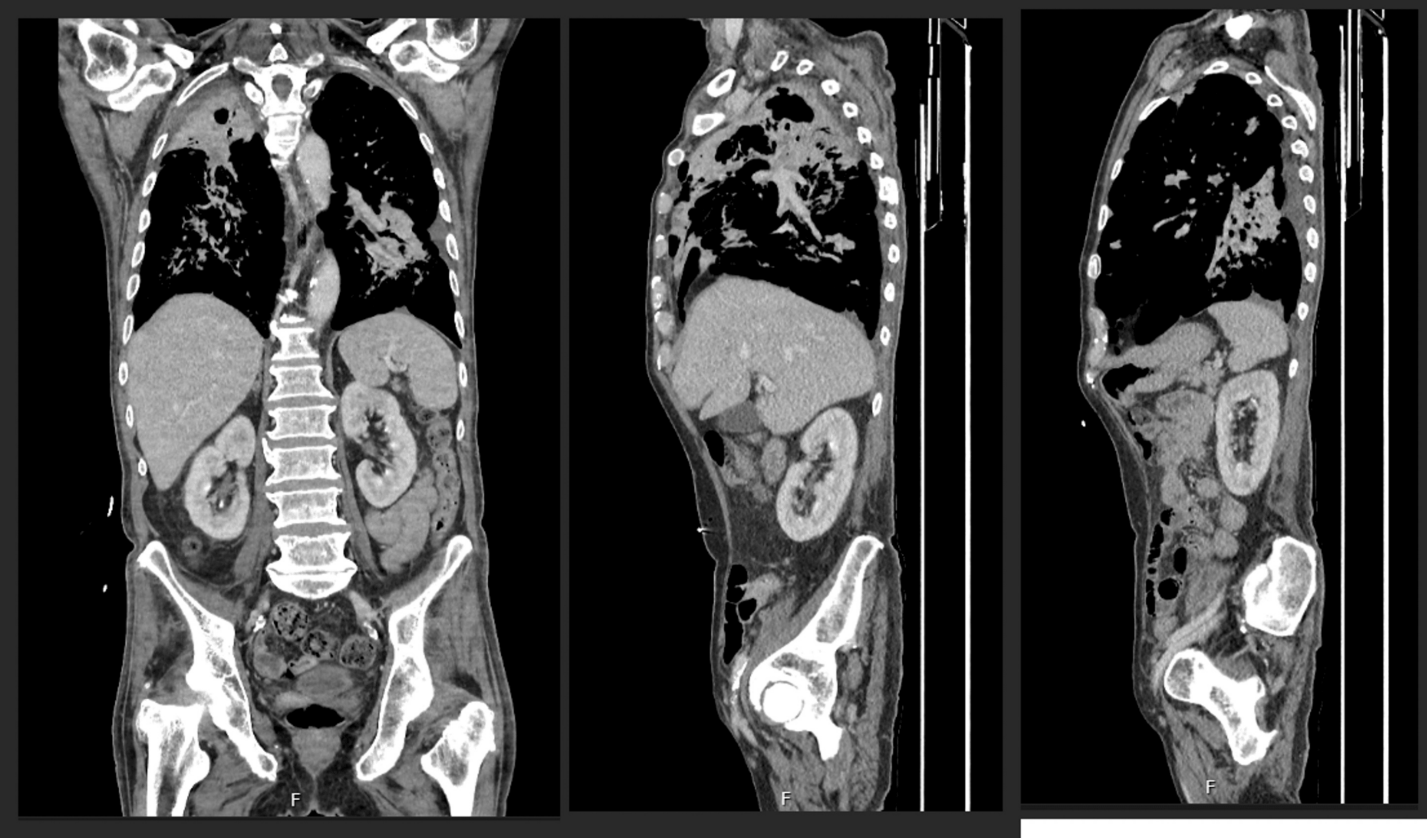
CT chest, abdomen, and pelvis showing stigmata of pulmonary TB and no signs of malignancy.
